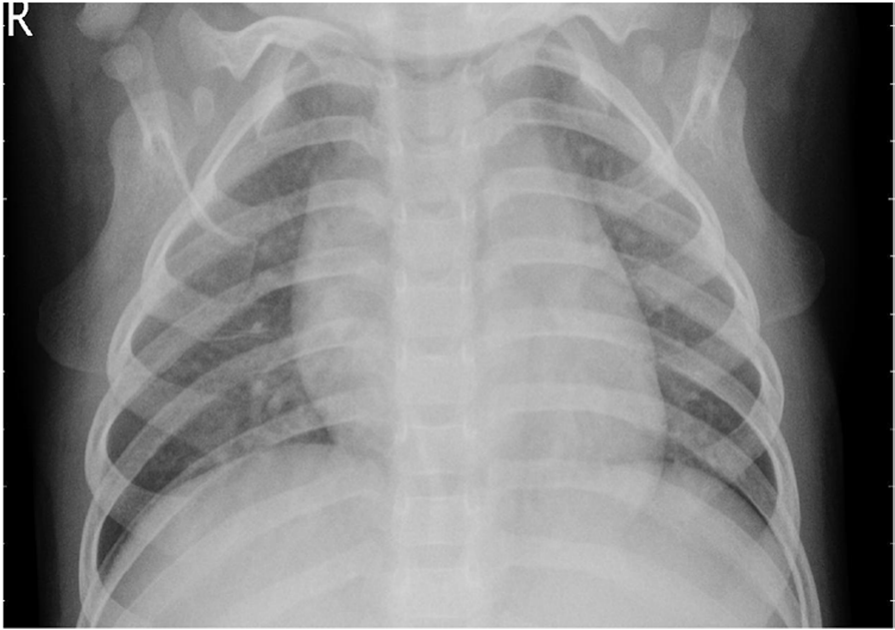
Sample of chest X-ray image of a healthy person
